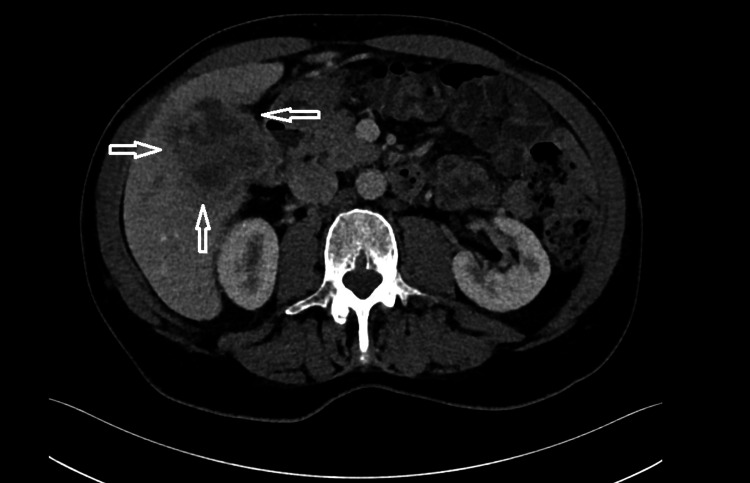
CT findings of the gallbladder tumor in coronal view. The arrows point out the tumor mass. CT: computed tomography
